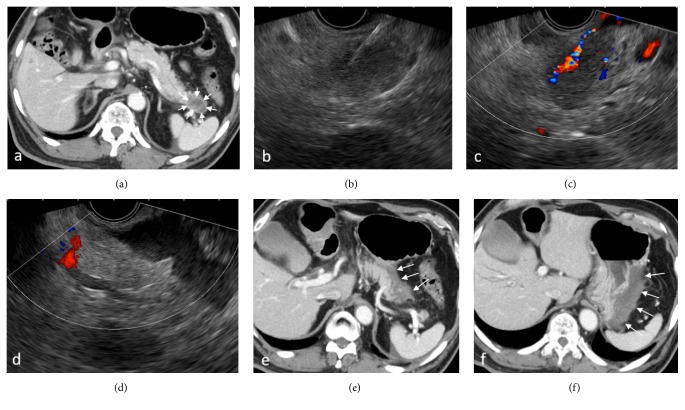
A patient who developed bleeding with endoscopic ultrasound-guided fine needle biopsy (EUS-FNB) using a Franseen needle. (a) Contrast-enhanced Computed Tomography (CT) scan showing a 3-cm hypovascular mass in the pancreatic tail (arrow). (b) Insertion of the needle under EUS guidance. (c) Active bleeding from the needle tract right was noticed under color Doppler mode after the withdrawal of the needle. (d) Increased echo-free space between the pancreas and stomach was identified. ((e), (f)) Contrast-enhanced CT scan was performed immediately after EUS-FNB. Hyperdense fluid collection suggesting hematoma was observed between the pancreatic tail and the greater curvature of the stomach (arrow).
